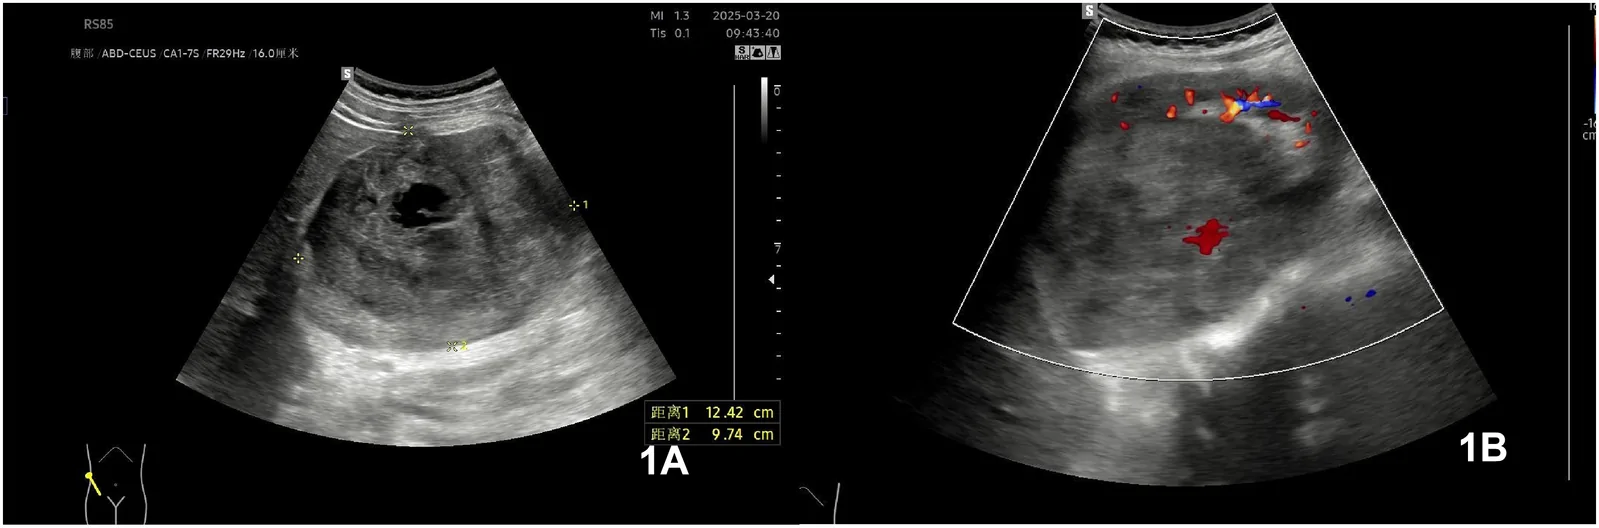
(A, B) Male, 56 years old, Ewing-like undifferentiated small round cell neoplasm of the kidney. Ultrasound examination (A) shows a hypoechoic mass in the right renal parenchyma, approximately 12.6 * 10.0 cm in size, with an unclear boundary, an irregular shape, and uneven internal echo. Color Doppler flow imaging (CDFI): A small amount of blood flow signal can be seen within the mass (B).
